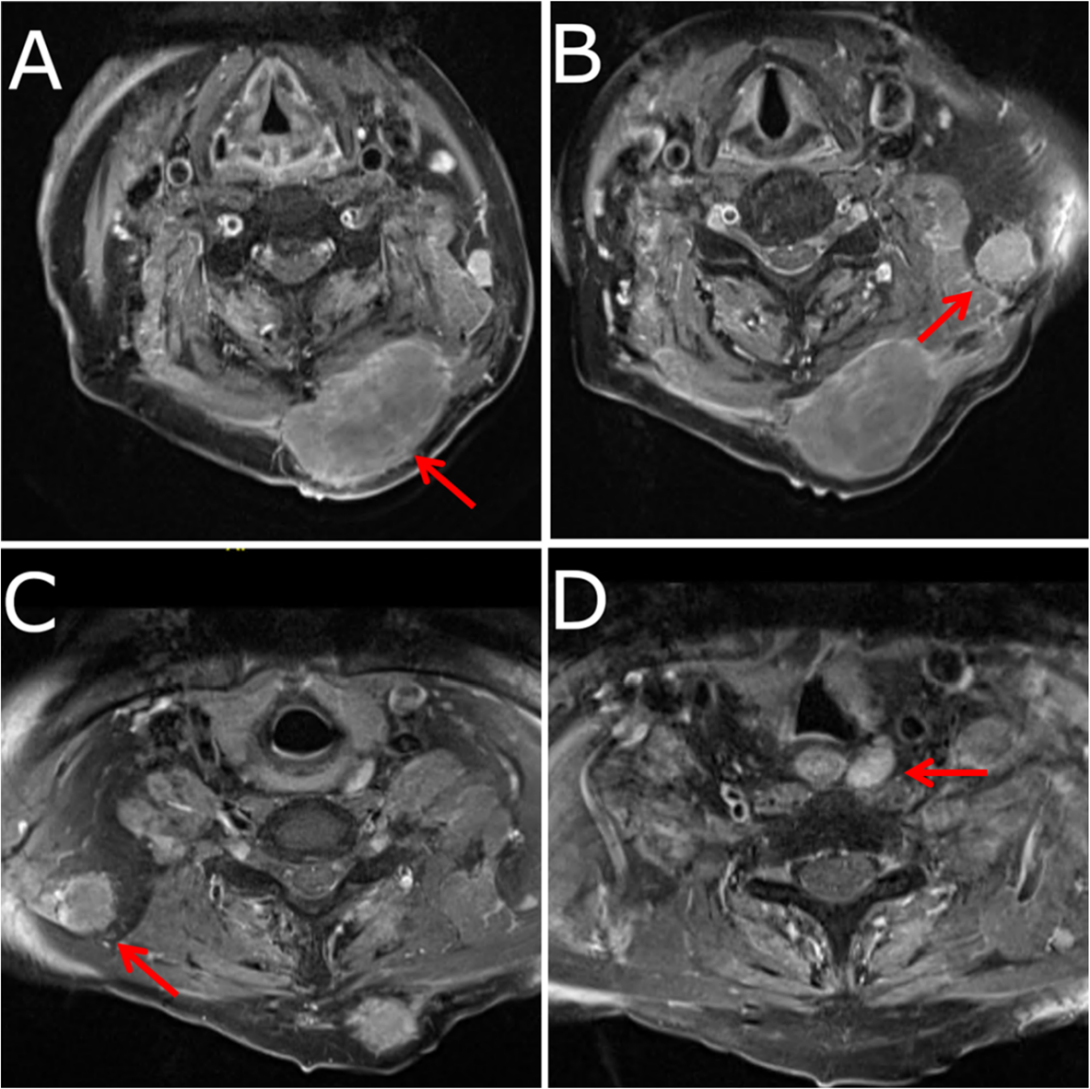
Four magnetic resonance images demonstrating metastatic disease throughout the soft tissue of the patient’s neck. A The clinically palpable lesion is shown located left of the midline of the posterior neck. B A lesion is shown located in the left posterior neck at level Vb. C A lesion is shown located in the right posterior neck at level Vb. D A lesion is shown located on the left side of the paraesophageal region at level VI
